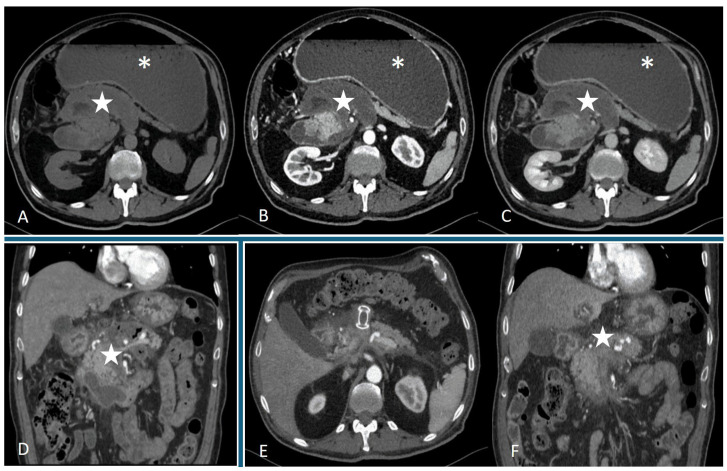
Axial unenhanced (A), arterial (B) and portal (C) phase CT images showing a known peripancreatic fluid collection (star) with multiloculated morphology and heterogeneous density, surrounded by thin walls, consistent with sequelae of recent necrotizing hemorrhagic pancreatitis. The collection measures approximately 12 × 8 cm in axial dimensions and 11 cm in craniocaudal extension, encasing the branches of the celiac trunk and the spleno-mesenteric-portal confluence. Associated marked gastric dilatation (asterisk) is also noted. Coronal arterial phase (D) showing that the collection (star) begins to exhibit imaging features consistent with the evolution toward walled-off necrosis (WON), including progressive wall maturation, internal non-liquid components such as necrotic debris, and the presence of gas bubbles. Following endoscopic drainage using a transgastric lumen-apposing metal stent (LAMS), axial (E) and coronal (F) arterial phase CT images demonstrate a marked reduction in the size of the collection (star), consistent with effective post-drainage evolution.
